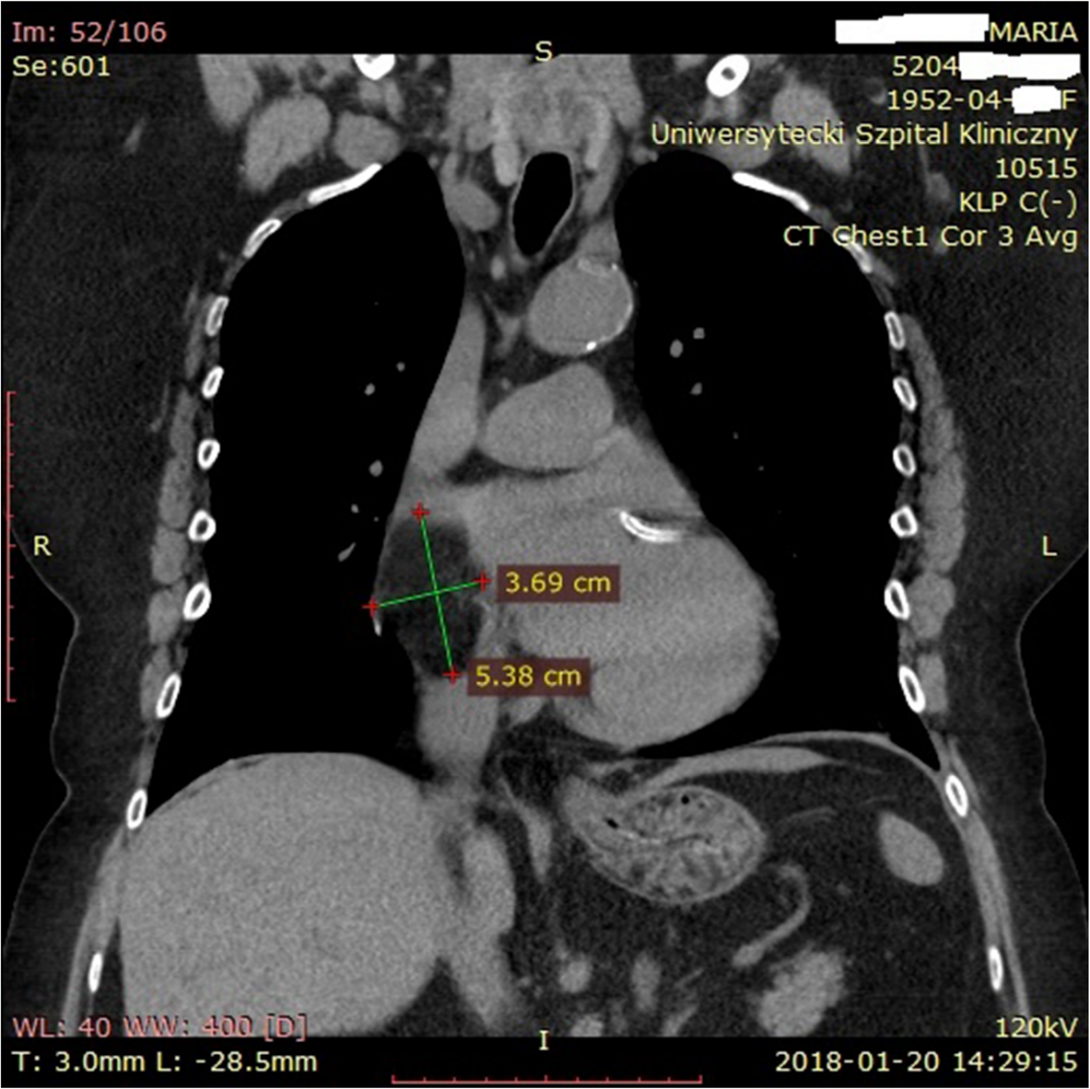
CT scan. Lipomatous mass in the interatrial septum (IVS) bulging to the right atrium (RA), in the axis of the SVC and IVC, measuring 3.69 cm × 5.38 cm
